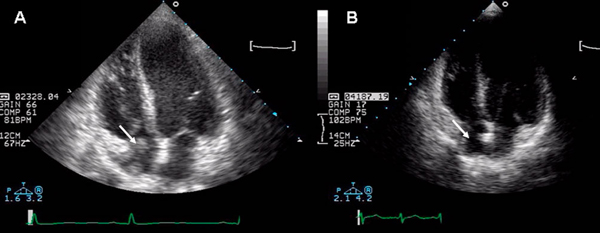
Four chamber view echocardiogram - pre (A) and post (B) treatment - showing resolution of the thrombus (arrow).
